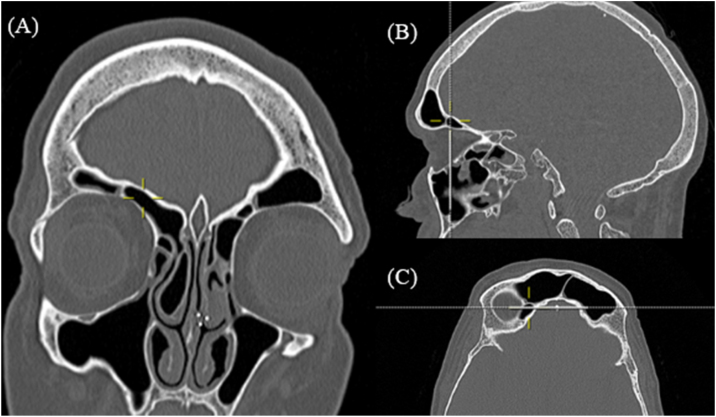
Supraorbital ethmoidal cell. Computed Tomography: yellow mark shows right supra supraorbital ethmoidal cell in coronal (A), sargital (B) and axial(C) reconstructions.
